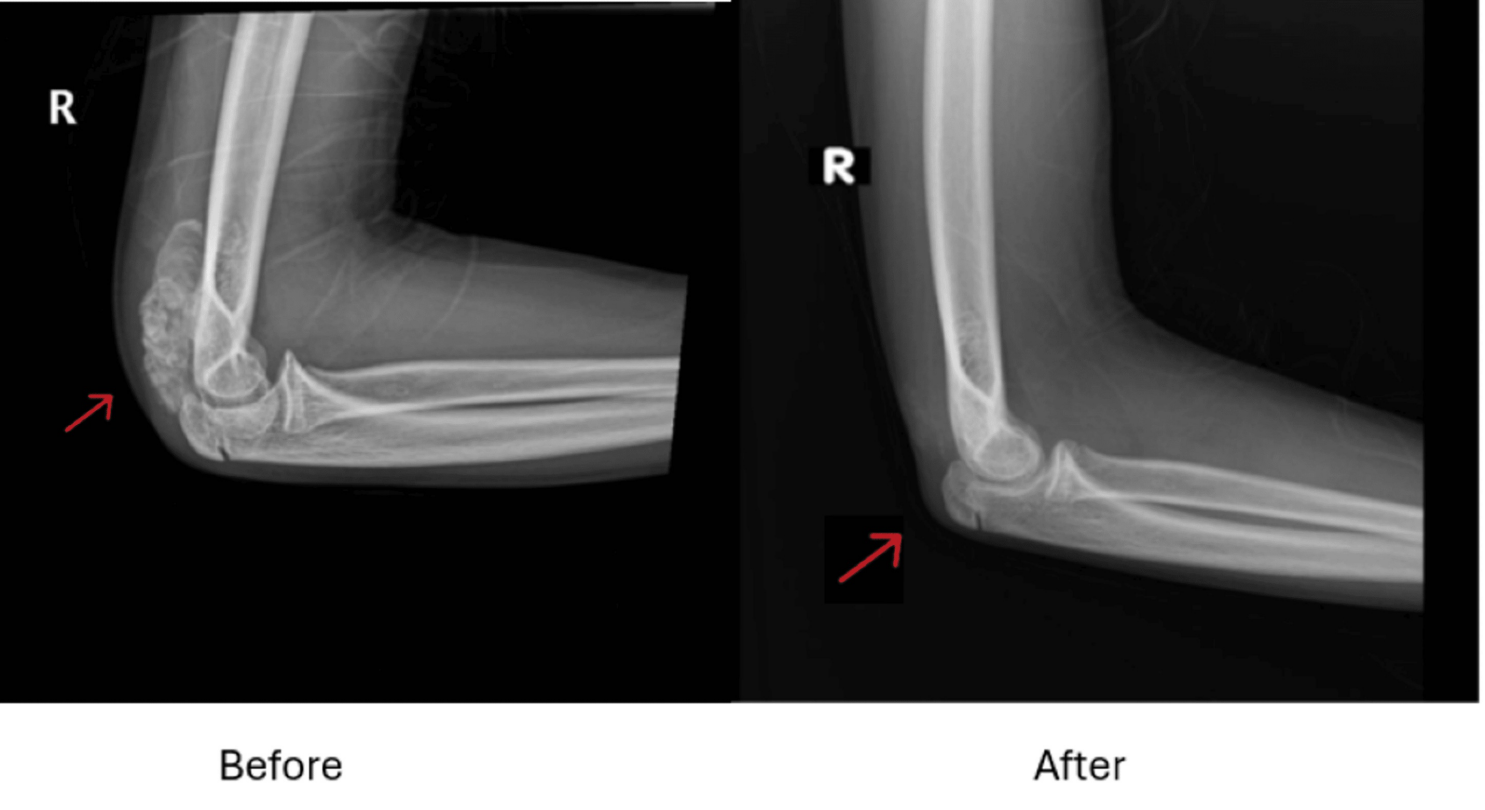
X-ray of Right Elbow Before and After Treatment for Hyperphosphatemic Familial Tumoral Calcinosis The "Before" image shows significant calcific deposits near the olecranon process of the right elbow (red arrow), characteristic of tumoral calcinosis. The "After" image demonstrates a marked reduction in the calcific mass following treatment, highlighting the efficacy of the intervention in reducing phosphate-related calcific deposits.
